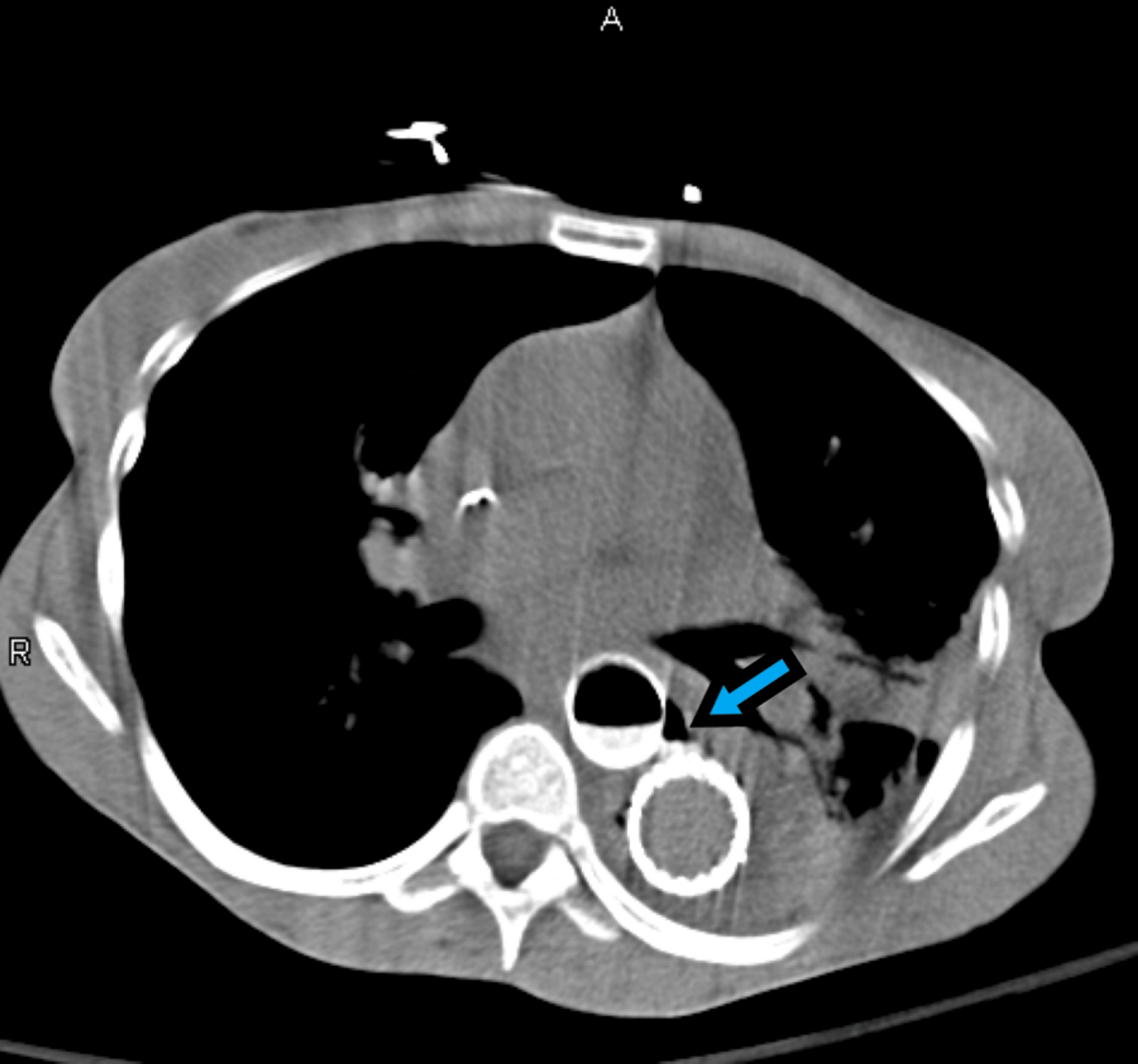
Computed tomography esophagram, axial plane, demonstrating communication between the esophagus and the false lumen of the thoracic aorta status post esophageal stent and thoracic endovascular aortic repair.
